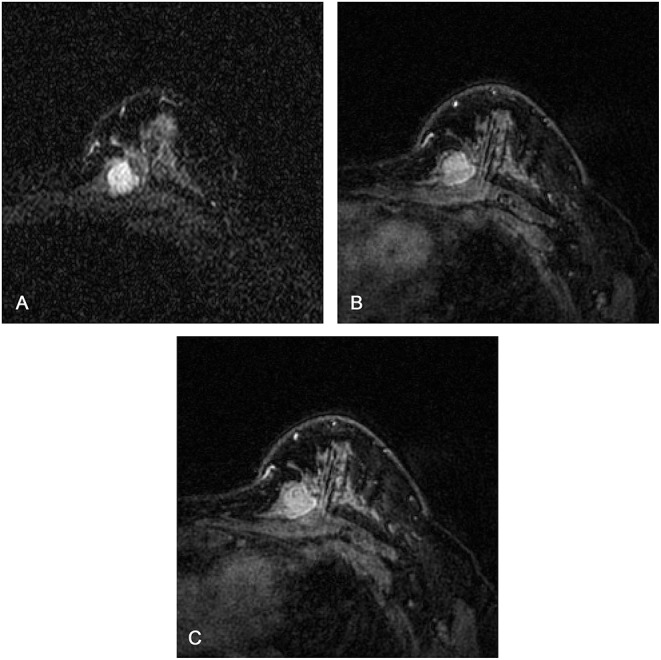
Magnetic resonance images of a 37-year-old female patient with a borderline phyllodes tumor: (A) axial short TI inversion recovery T2-weighted (T2W-STIR) (B) first post-contrast (C) third post-contrast. The texture features on T2W-STIR correctly identified a phyllodes tumor which was falsely interpreted as a fibroadenoma on clinical and conventional MRI features, possibly owing to the weak lobulation, homogeneous signal on T2W-STIR, and absence of cystic component and septation.
